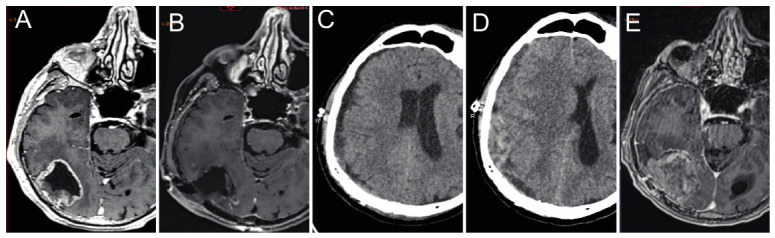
Representative images (MRI and CT scan) at different timepoints during patient’s management. (A) Preoperative T1 MRI with gadolinium injection showing a right occipital contrast-enhancing lesion with necrotic center and perilesional edema. (B) Postoperative T1 MRI with gadolinium injection confirming total resection of the contrast-enhancing component of the lesion without postoperative complication. (C) Postoperative CT scan after the evacuation of the first right-sided chronic SDH. (D) Emergency CT scan showing the recurrence of the right-sided SDH and its mass effect on the parenchyma (E) T1 MRI with gadolinium injection showing the recurrence of the right occipital lesion in the initial resection site.
